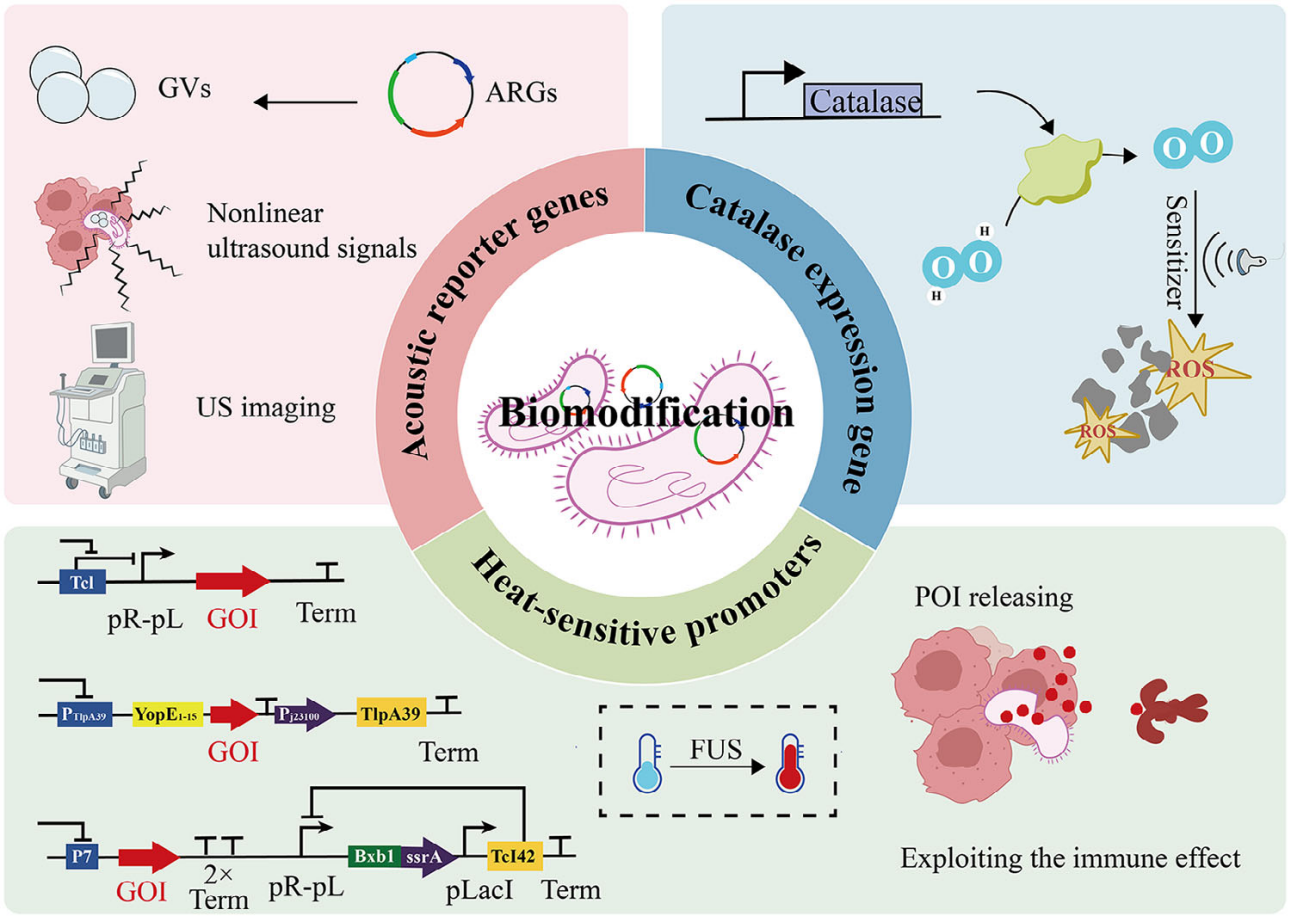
Biomodification strategies of ultrasound‐responsive bacteria. Reproduced with permission. [15] Copyright 2022, Springer Nature. Reproduced with permission. [17] Copyright 2024, Elsevier. Reproduced with permission [16]. Copyright 2022, Springer Nature.
